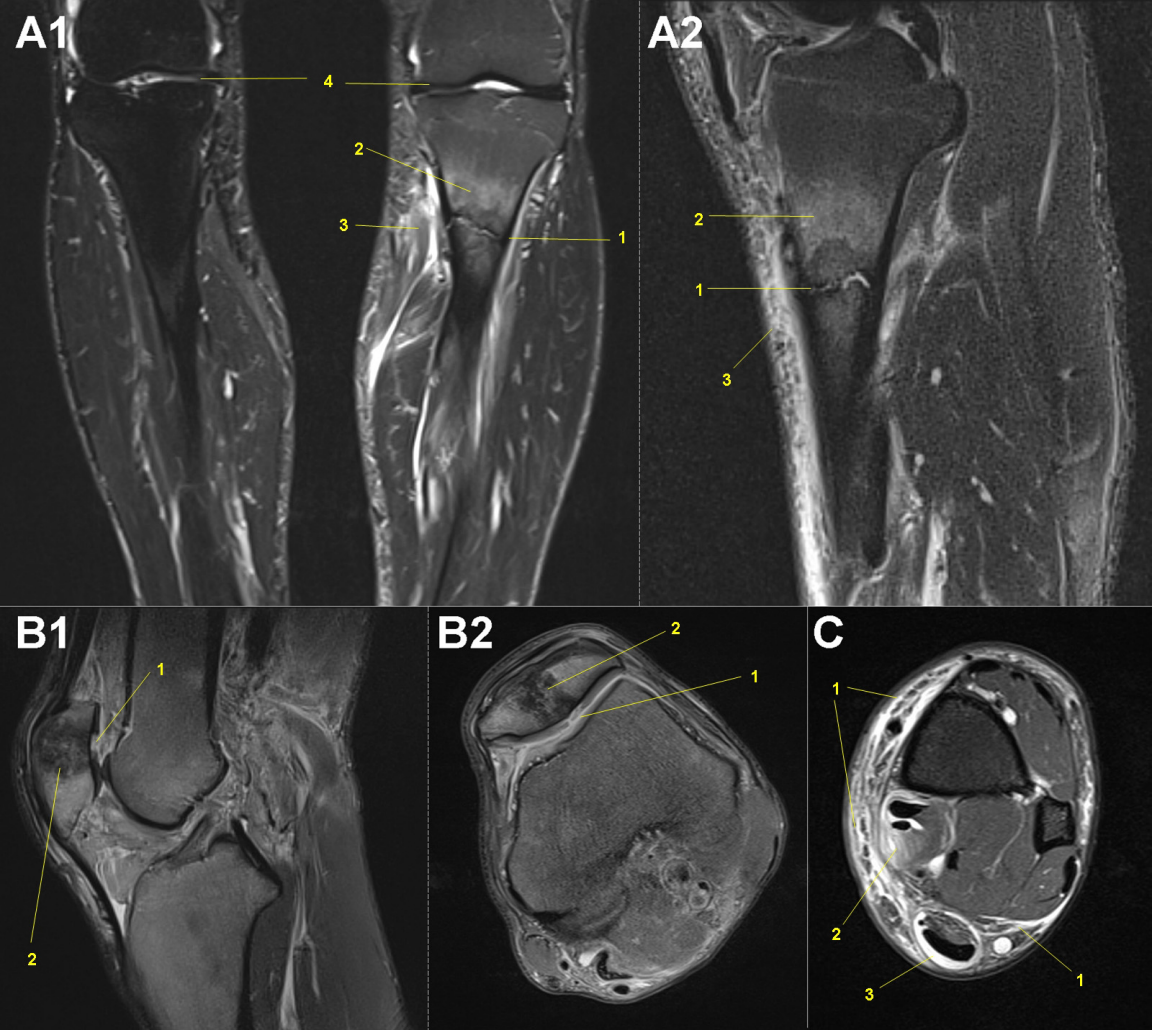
Supplementary mobile MRI examinations during the TEFR09. A: male, 61-years-old, stage 38, 2,601 km (PDw TSE fs, A1: coronal, A2: sagittal): Stress fracture of the proximal tibia (1) with perifocal bone edema (2) and focal subcutaneous edema (3). B: male, 49-years old, stage 52, 3,609 km (PDw TSE fs, B1: sagittal, B2: transversal): Retropatellar chondral ulcer (1) leading to bleeding/hematoma of the patellar bone (2). C: male, 41-years-old, stage 13, 857 km (PDw TSE fs transversal): Massive subcutaneous edema (panniculitis: 1) with focal myositis of deep flectors (2) and tenosynovitis of Achilles tendon (3).
